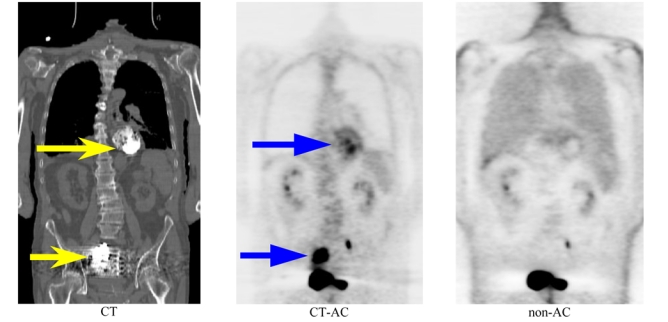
Oral contrast-enhanced related artefact in clinical PET/CT imaging. The region concentrating oral contrast shown on CT (left, arrows) led to areas of apparently increased glucose metabolism on CT-based attenuation corrected PET (centre, arrows). On fused PET/CT images, this area of apparently increased glucose metabolism correlated with high-density oral contrast on CT (not shown). Reconstructed PET images without attenuation correction demonstrated absence of lesions (right), demasking areas of apparently increased glucose metabolism as artefact. Courtesy of Prof. H. Abdel-Dayem.
